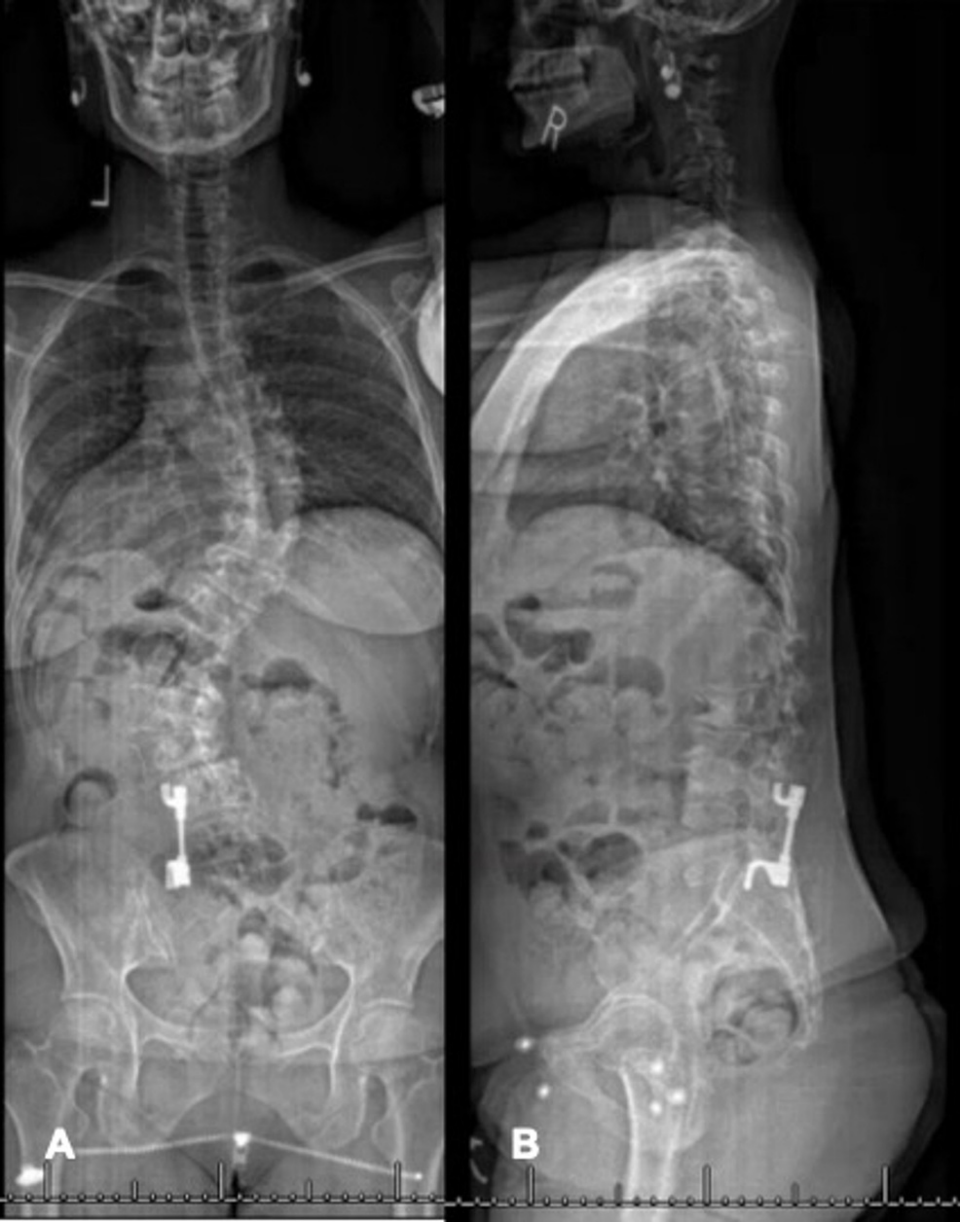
(A) AP and (B) lateral scoliosis X-ray of unilateral L4-sacrum instrumentation for treatment of adolescent idiopathic scoliosis. AP- anteroposterior
